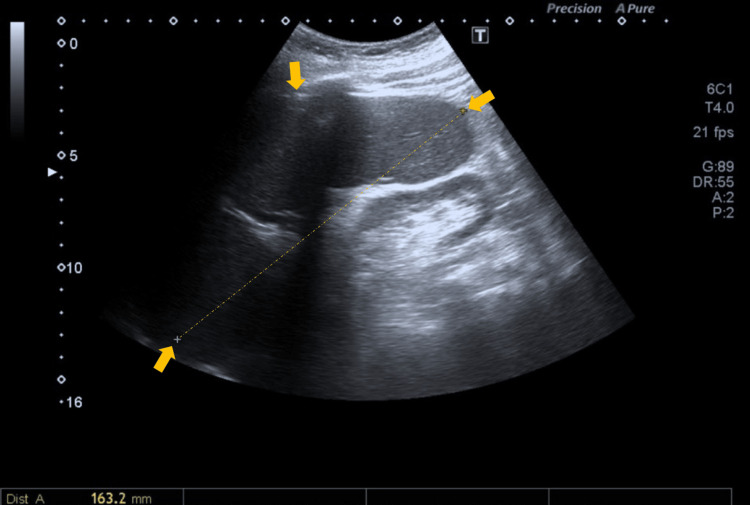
Abdominal ultrasound revealing splenomegaly The yellow arrows indicate splenomegaly, and the line indicates the spleen length
